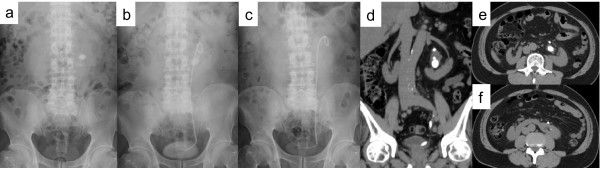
Case 1: (a) preoperative kidneys, ureters, and bladder X-ray films, (b) preoperative stenting, and (c) postoperative. Preoperative non-contrast (d) coronal and (e, f) axial computed tomography images.
